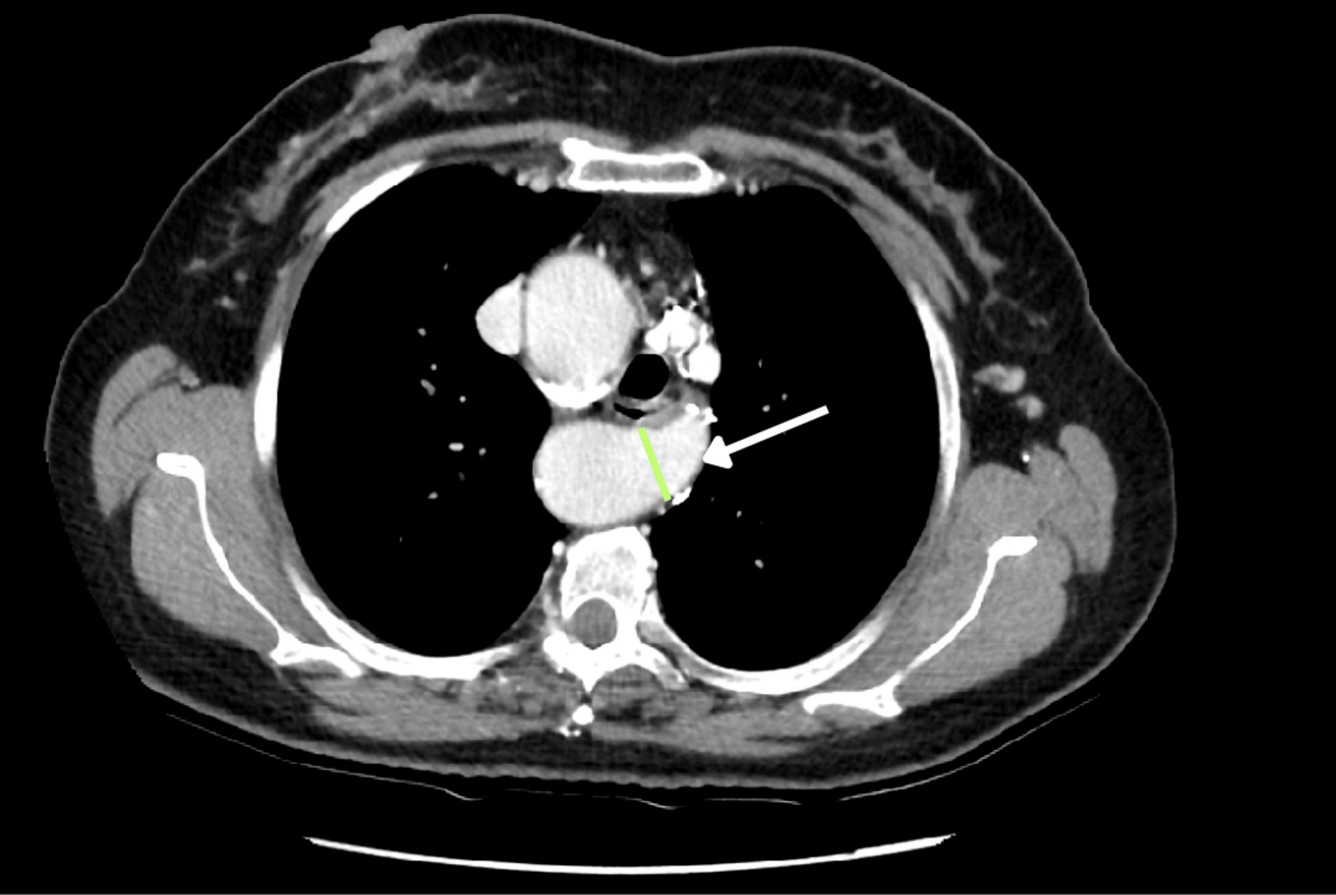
Axial view of the CT scan showing KD (green arrow) and the left aberrant subclavian artery (white arrow) KD: Kommerell’s diverticulum
